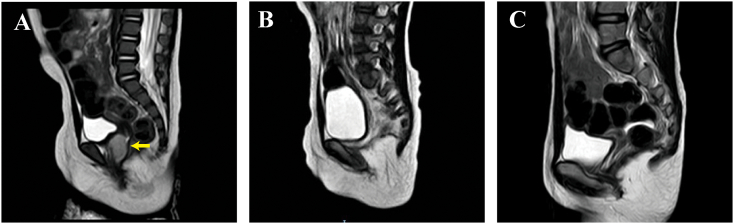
Sagittal MRI of the pelvis before and after chemotherapy. A) Before chemotherapy, a round and well-defined vaginal mass (arrow) was observed. B) After 3 cycles of chemotherapy, no significant vaginal tumor was detected. C) Follow-up MRI at 60 months revealed no evidence of a mass in the anterior vagina.
